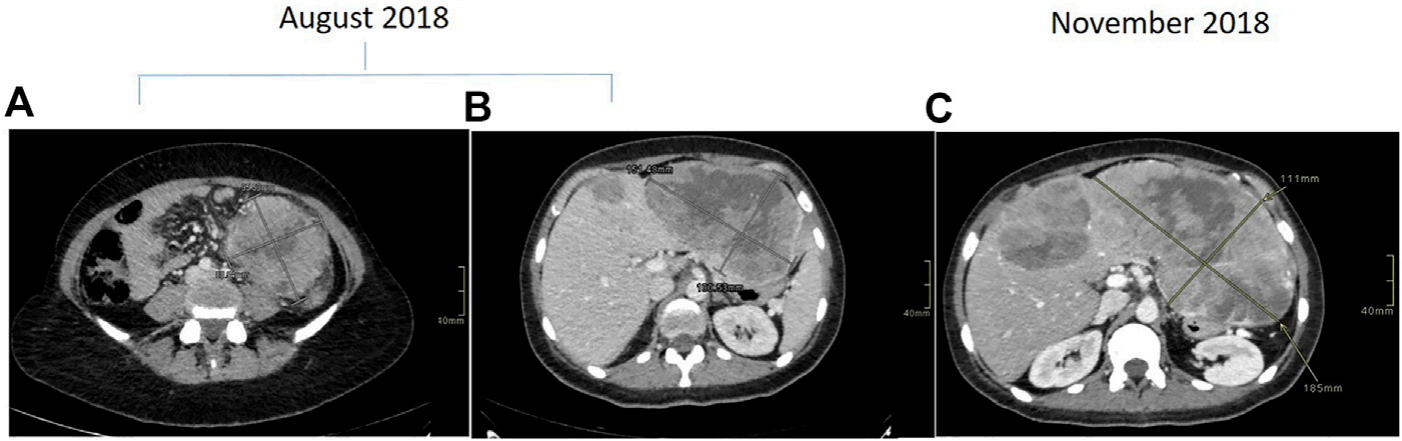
Contrast enhanced CT scan demonstrating descending colon cancer at baseline (A) and liver metastasis at baseline (B) and at progression (C).
